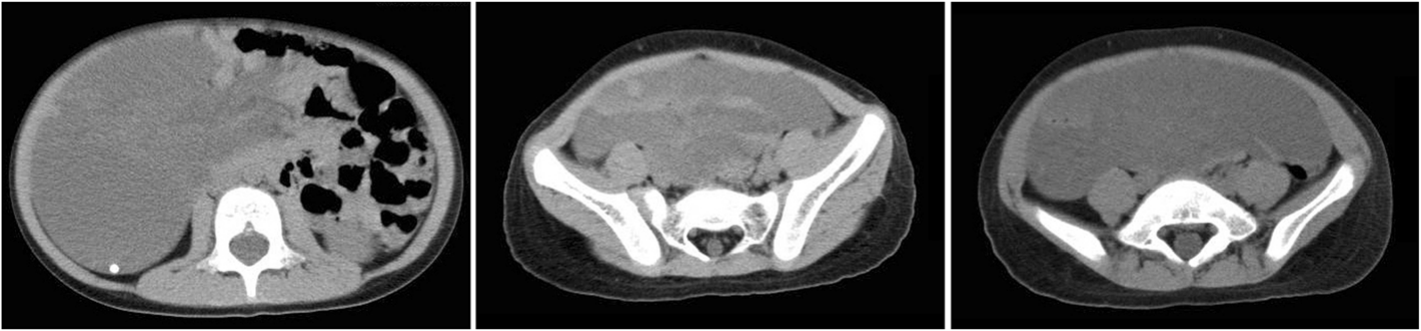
CT demonstrated a giant solid-cystic abdominal mass with heterogeneous density, which was mainly composed of cystic elements.
